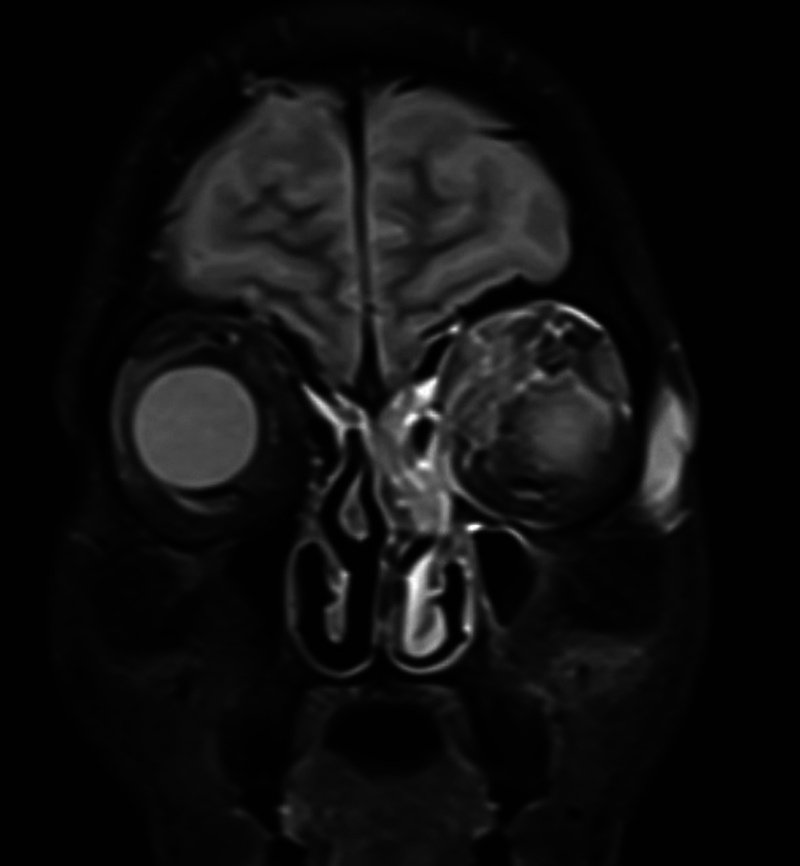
Magnetic resonance imaging T2 fat sat coronal view shows hyperintense signal within Medial superior orbital compartment up to the superior rectus pushing left globe inferolaterally with hyperintense signal within bony lateral orbital margin (zygomatic bone affection) – prominent left ophthalmic vein.
